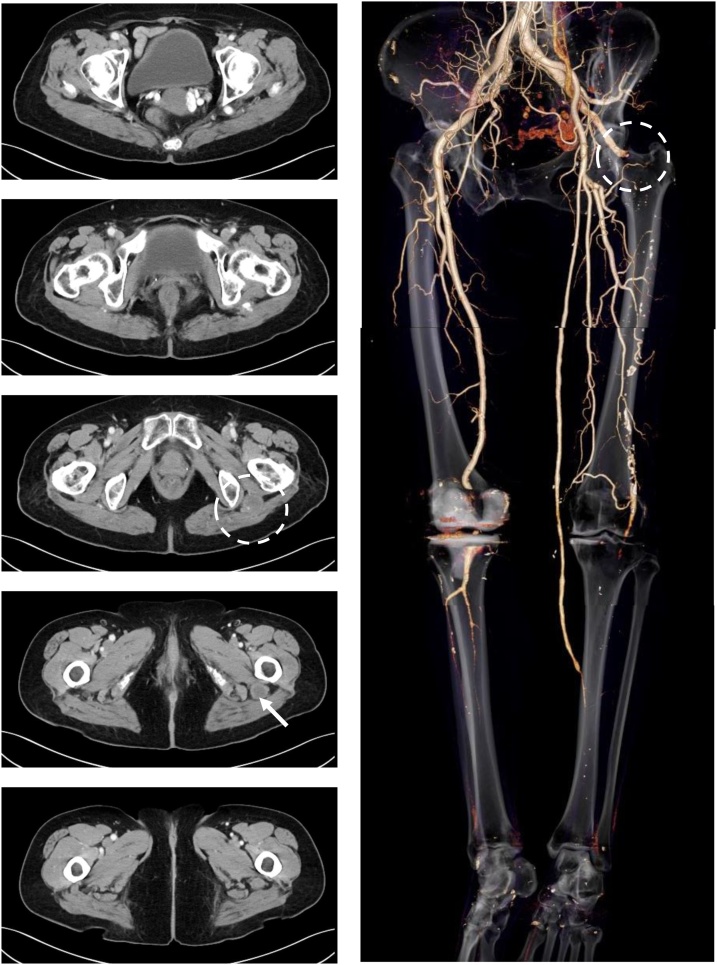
Enhanced CT images one year after the surgery. The bypass graft is patient and the artery from sciatic to superficial femoral is occluded at the PSA aneurysm (circle). The dilatation of PSA aneurysm has not been observed, its size is 22 mm in maximum minor-axis diameter (arrow).
